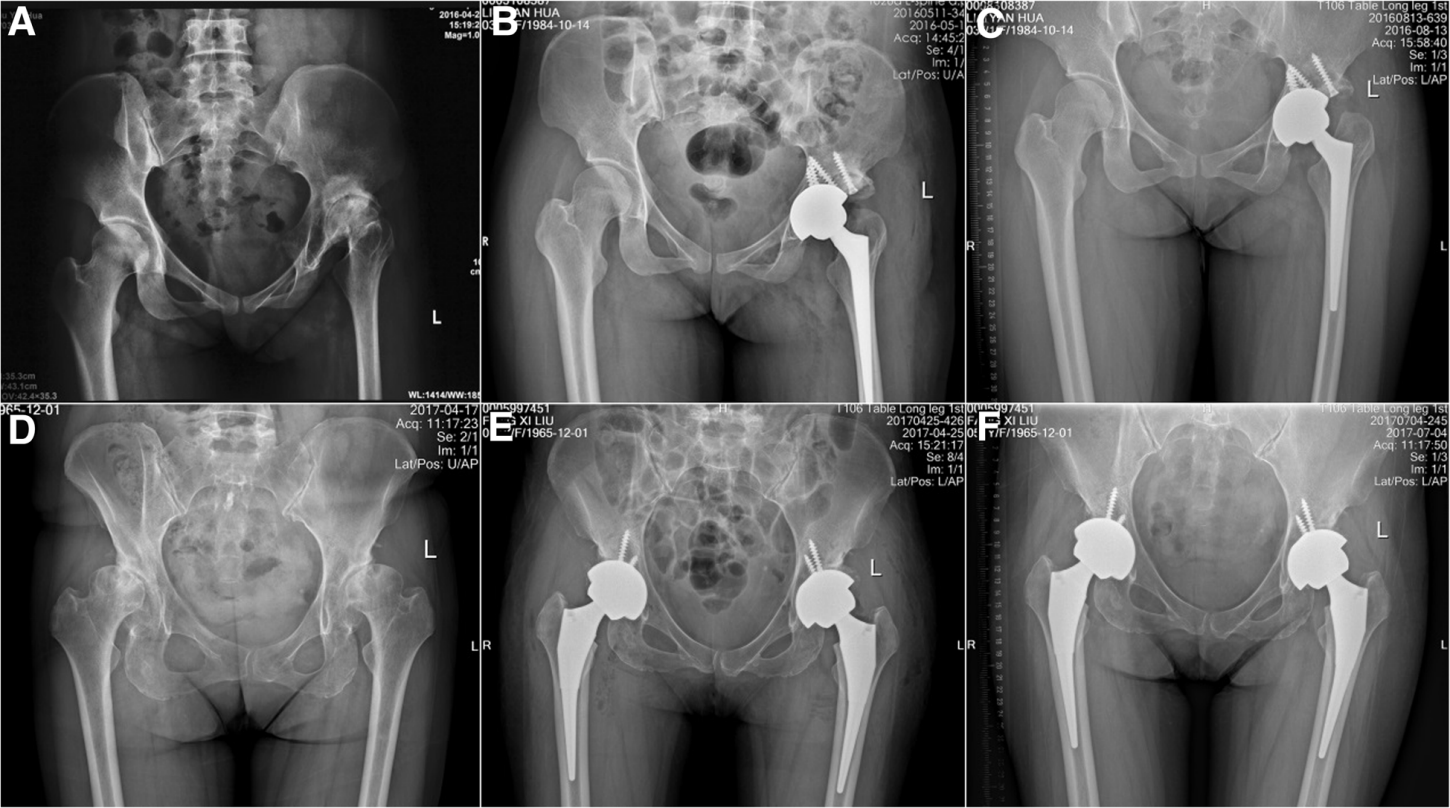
Samples from our patients. a The preoperative X-ray of patient 1: a 32-year-old female diagnosed with the Crow type III DDH of the left hip. b The postoperative X-ray of patient 1 (2 days after surgery): the designed inclination was 45° (left), whereas the anteversion was 15° (left). The postoperative inclination was 48.3° (left), whereas the anteversion was 18.4° (left). c The postoperative X-ray of patient 1 (3 months after surgery): the acetabular components appeared radiologically stable. d The preoperative X-ray of patient 2: a 51-year-old female diagnosed with the Crow type II DDH on both sides of the hips. e The postoperative X-ray of patient 2 (4 days after surgery): the designed inclination values were 46° (left) and 45° (right), whereas the anteversion values were 10° (left) and 18° (right). The postoperative inclination values were 48.7° (left) and 41.8° (right), whereas the anteversion values were 7° (left) and 21.6° (right). f The postoperative X-ray of patient 2 (3 months after surgery): the acetabular components appeared radiologically stable
